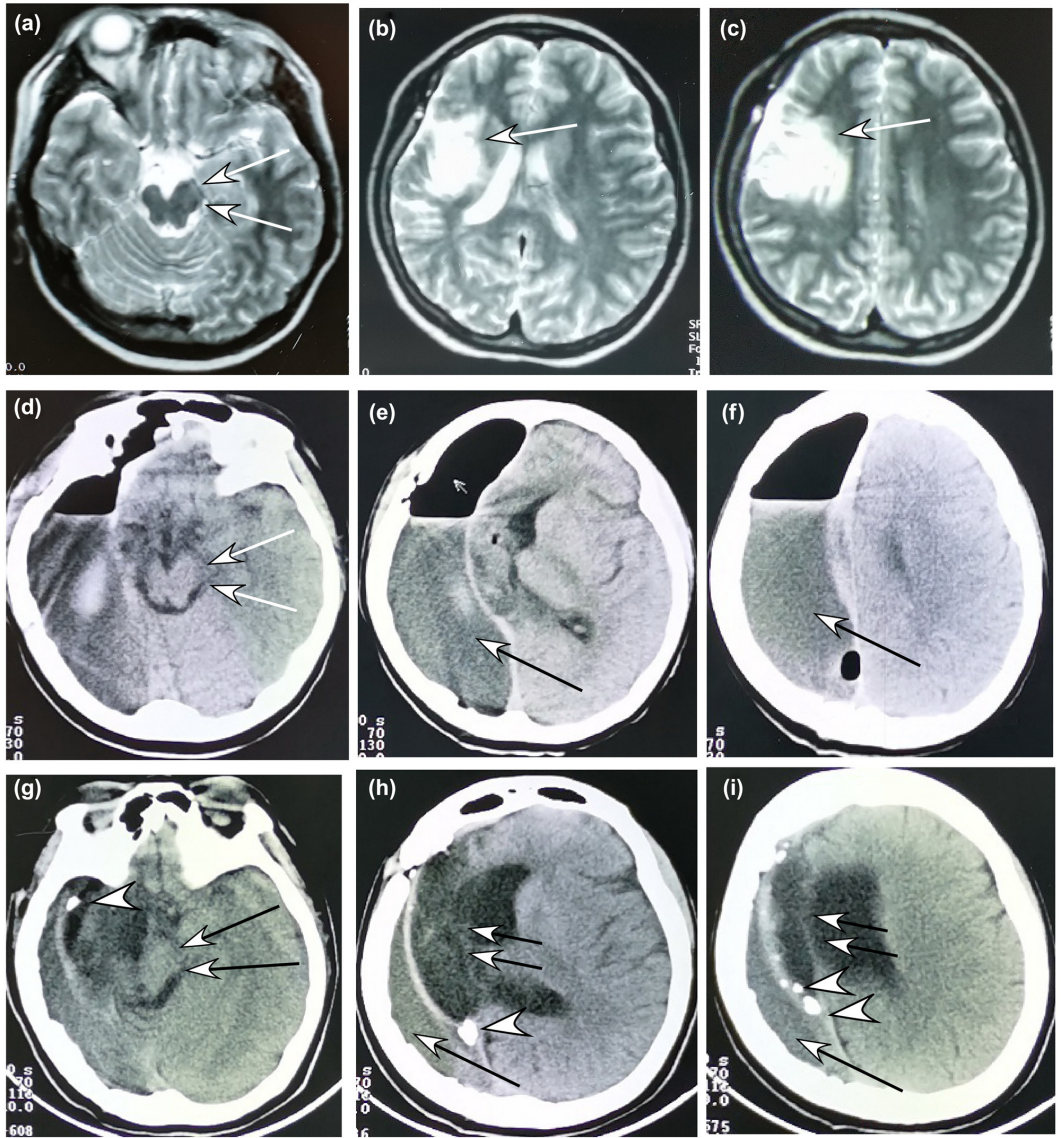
A 16-year-old girl had medically refractory epilepsy and underwent hemispherectomy. (a–c) Before the hemispherectomy, magnetic resonance imaging of the brain revealed compensatory enlargement of the left cerebral peduncle but atrophic right cerebral peduncle (arrow in A) and atrophic brain tissue (arrow in B and C). (d–f) Computed tomographic scan (CTS) at 7 days posthemispherectomy demonstrated enlarged cerebral peduncle (arrows in D) on the left side and a residual intracranial space (arrow in E and F) on the right side with accumulation of air and fluid. (g–i) CTS 3 years later showed enlarged cerebral peduncle on the left side (double arrows in G), right-shifted center line (double arrows in H and I), and shrank epidural space (bigger arrow in H and I) under the skull. Calcification (arrow head in G–I) occurred on the dura mater bordering the shrank epidural space and the left hemisphere, and this calcified dura mater gave a support to the left hemisphere.
